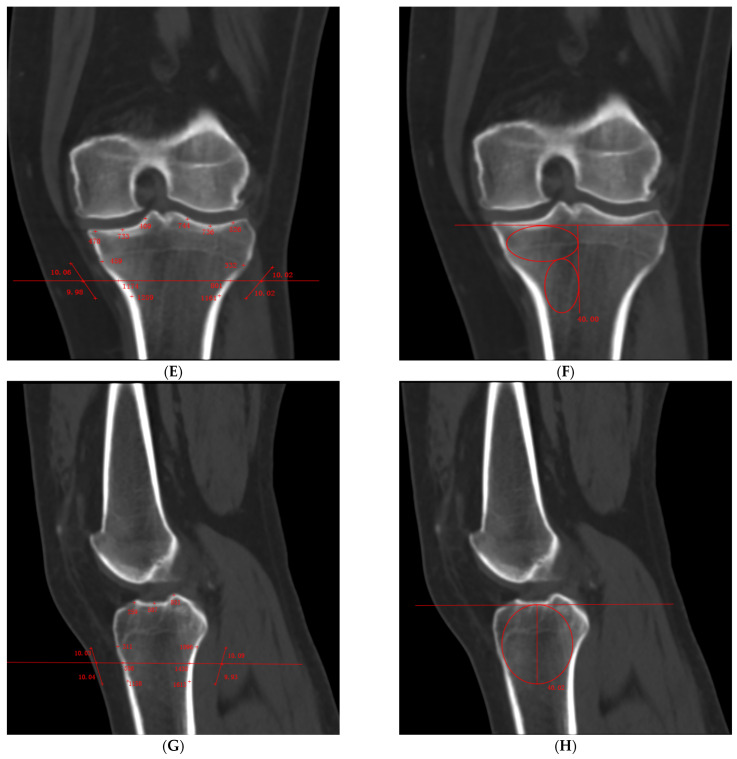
CT scan protocol. Abbreviations: HU, Hounsfield units; SP, sagittal plane; CP, coronal plane. (A) Cortical bone HU values in the SP of the femur, (B) lateral condyle cancellous bone HU values in the SP of the femur, (C) cortical bone HU values in the CP of the femur, (D) lateral condyle cancellous bone HU values in the CP of the femur, (E) cortical bone HU values in the CP of the tibia, (F) medial condyle cancellous bone HU values in the CP of the tibia, (G) cortical bone HU values in the SP of the tibia, (H) medial condyle cancellous bone HU values in the SP of the tibia.
